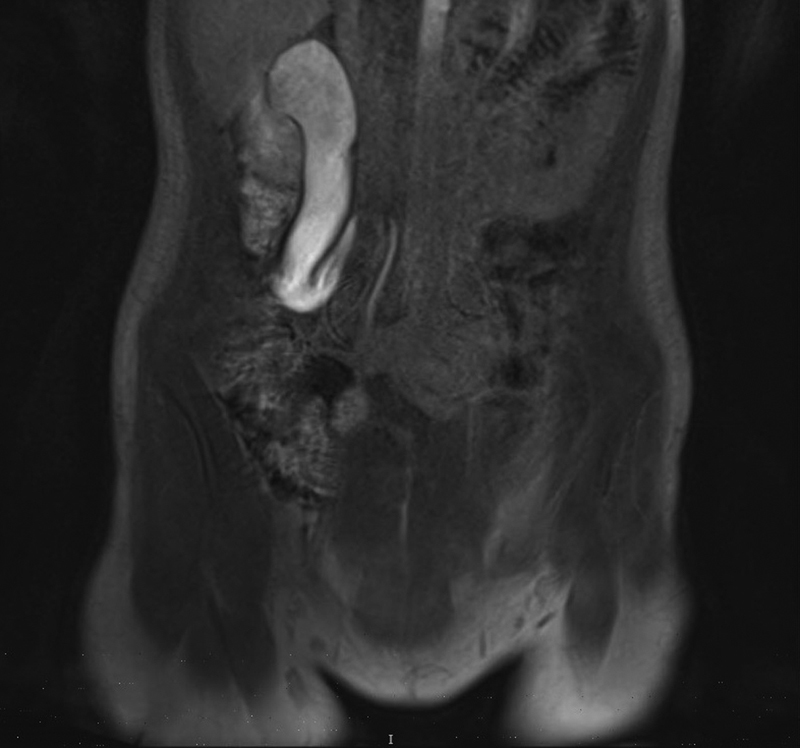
Coronal view of the magnetic resonance image of the typical type 1 retrocaval ureter variant with S-shaped deformity of right lumbar ureter with hydronephrosis and dilatation of the associated proximal ureter (case 2).
